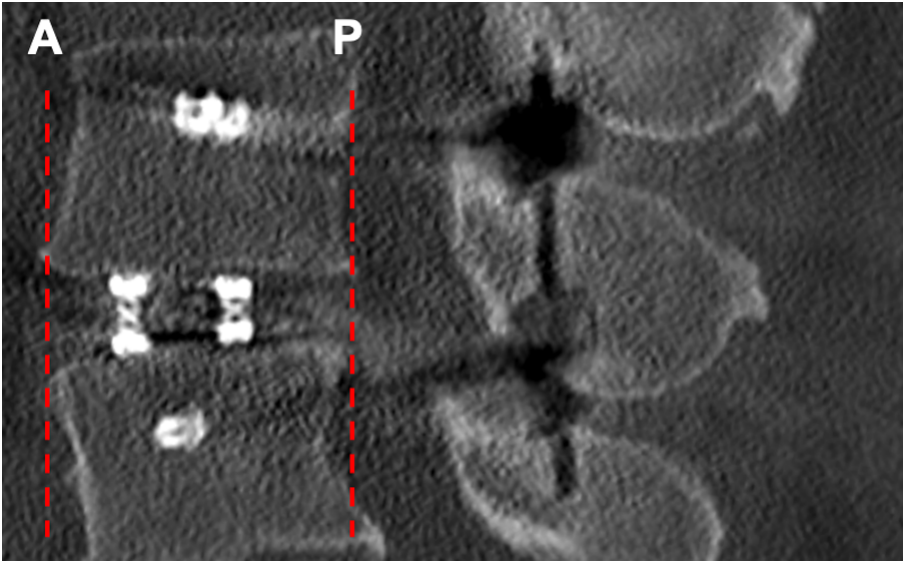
Radiographic evaluation of cage placement. Figure 2 shows an intraoperative CT-scan after 360-degree instrumentation of the lumbar spine in a sagittal view. Cage placement was evaluated in anterior (A) and posterior (*P*) orientation in relation to the limits of the upper and lower endplate of the adjacent vertebrae.
